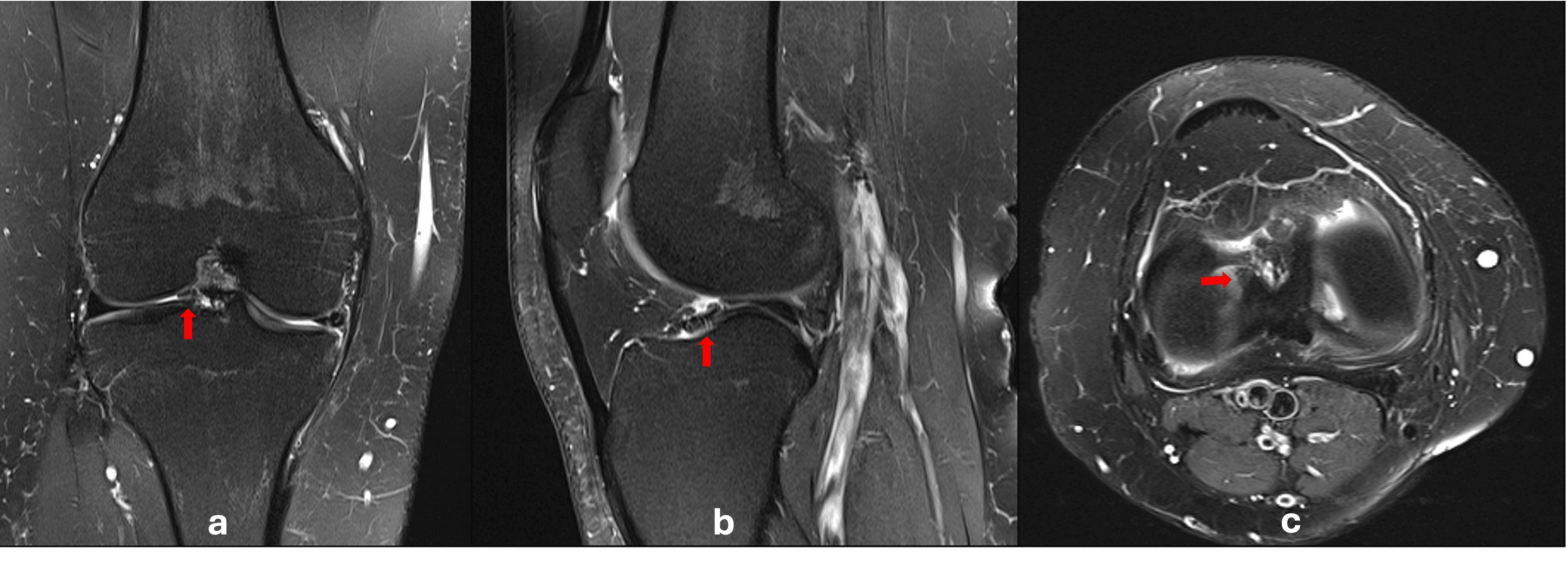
MR images showing the aberrant meniscal tissue (red arrow). (a) Coronal view demonstrating the inner portion of the ring-shaped meniscus, known as the "mirror image sign", a triangular structure mirroring the body of the meniscus. (b) Sagittal view showing "central bow tie sign" signifying a lateral meniscus fragment in the notch. (c) Axial view demonstrating a central portion or the intermeniscal bridge of the lateral meniscus fragment. MR: magnetic resonance
